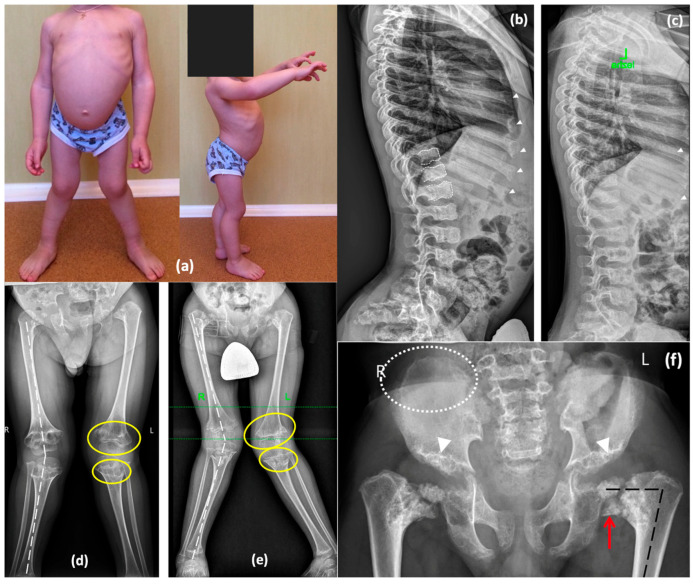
Phenotype and skeletal radiographies of P4 at various ages: (a) A photo taken at 3 years. Lateral radiographs of the spine at the age of 5 years (b) and 7 years (c)—double-convex contour of the endplates (white dotted lines) is moderately represented at the younger age and decreases later; enlarged distal parts of the ribs with rickets-like edges demonstrate the same pattern of improvement (white arrowheads). Lower limb radiographs at the age of 5 years (d) and 7 years (e) demonstrate progressive valgus deformities (white broken lines) secondary to the remarkable progression of the metaphyseal “chondromatous” changes (indicated by yellow lines). Pelvis radiographs at the age of 7 years (f) demonstrate coxa vara (black broken lines) secondary to the shortening and abnormal ossification of the femoral neck and head with Fairbank’s triangle (red arrow), dysplastic acetabulae (white arrowheads), and lace-like appearance of the iliac crests (rounded with the white dotted lines).
